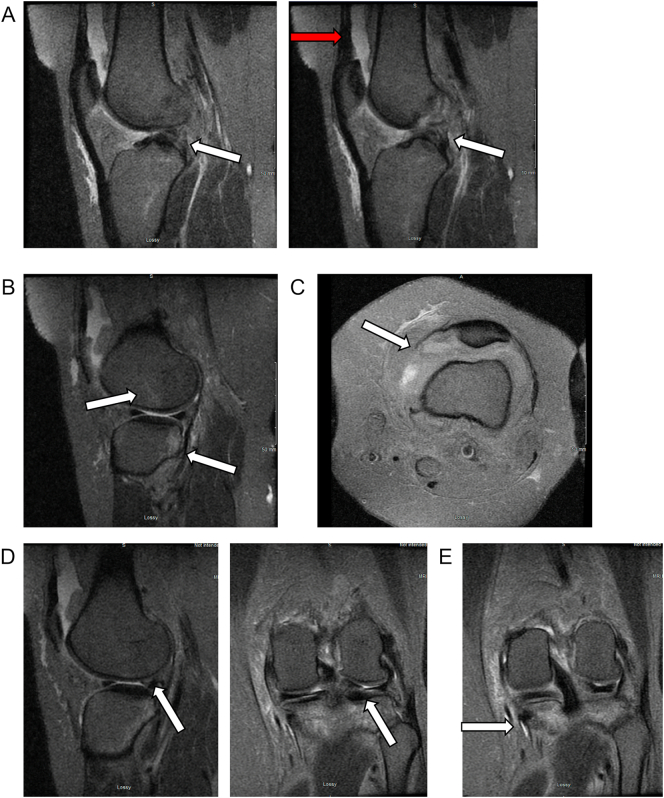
Sagittal cuts of magnetic resonance imaging (MRI) of the left knee demonstrating a vertical tear of the lateral meniscus, specifically the posterior horn and root of lateral meniscus extending through midportion. Moderate knee joint effusion is appreciated.
